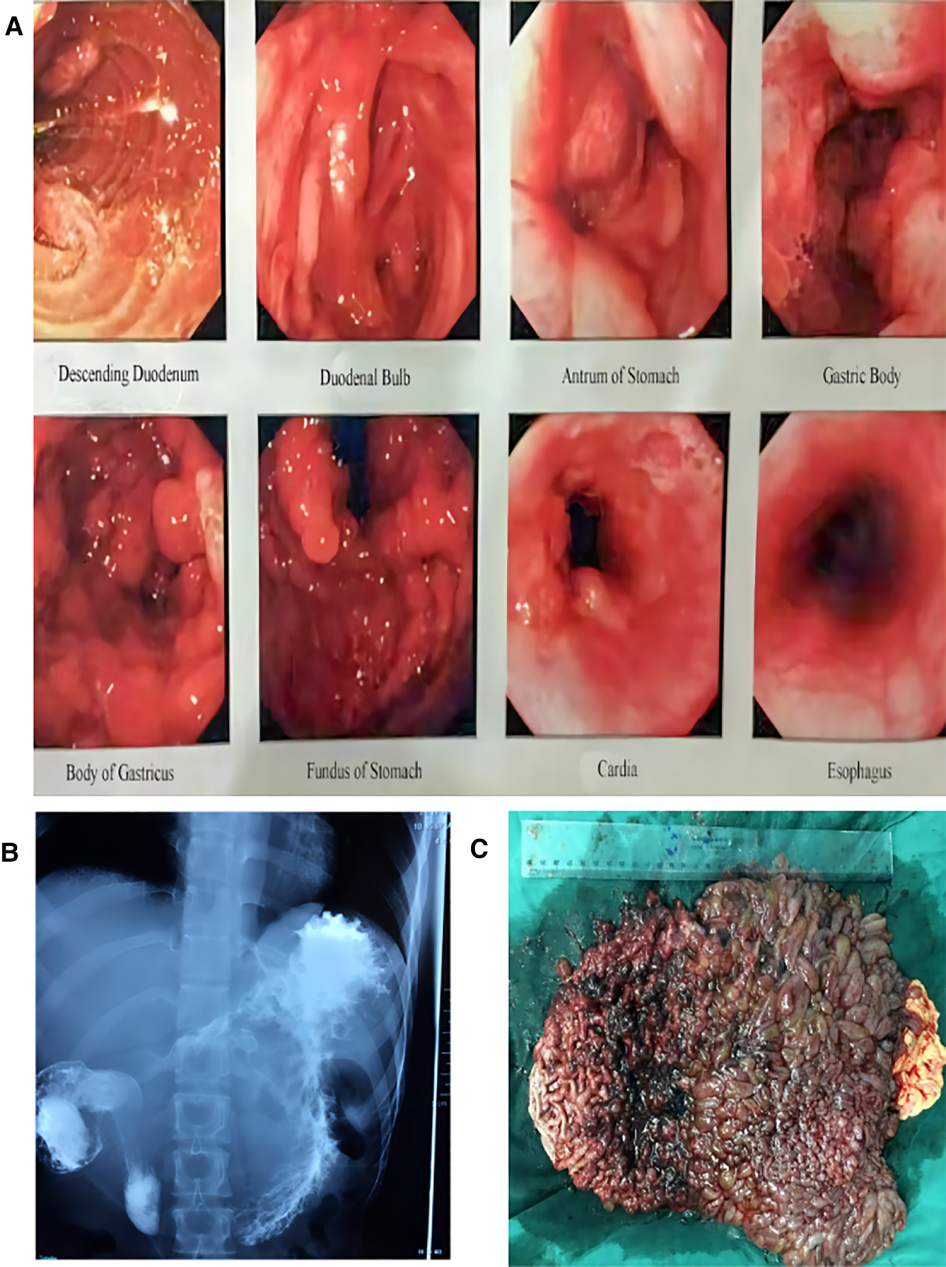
(A) Endoscopic findings. An upper endoscopy showing the diffuse benign inflammatory gastric polyps caused a subtotal obstruction of the gastric outlet. (B) UGBCR showed gastric mucosal destruction and a huge filling defect in the stomach. (C) The stomach is being filled with polyps. These polyps showed a coral plexiform change. UGBCR, upper gastrointestinal barium contrast roentgenography.
